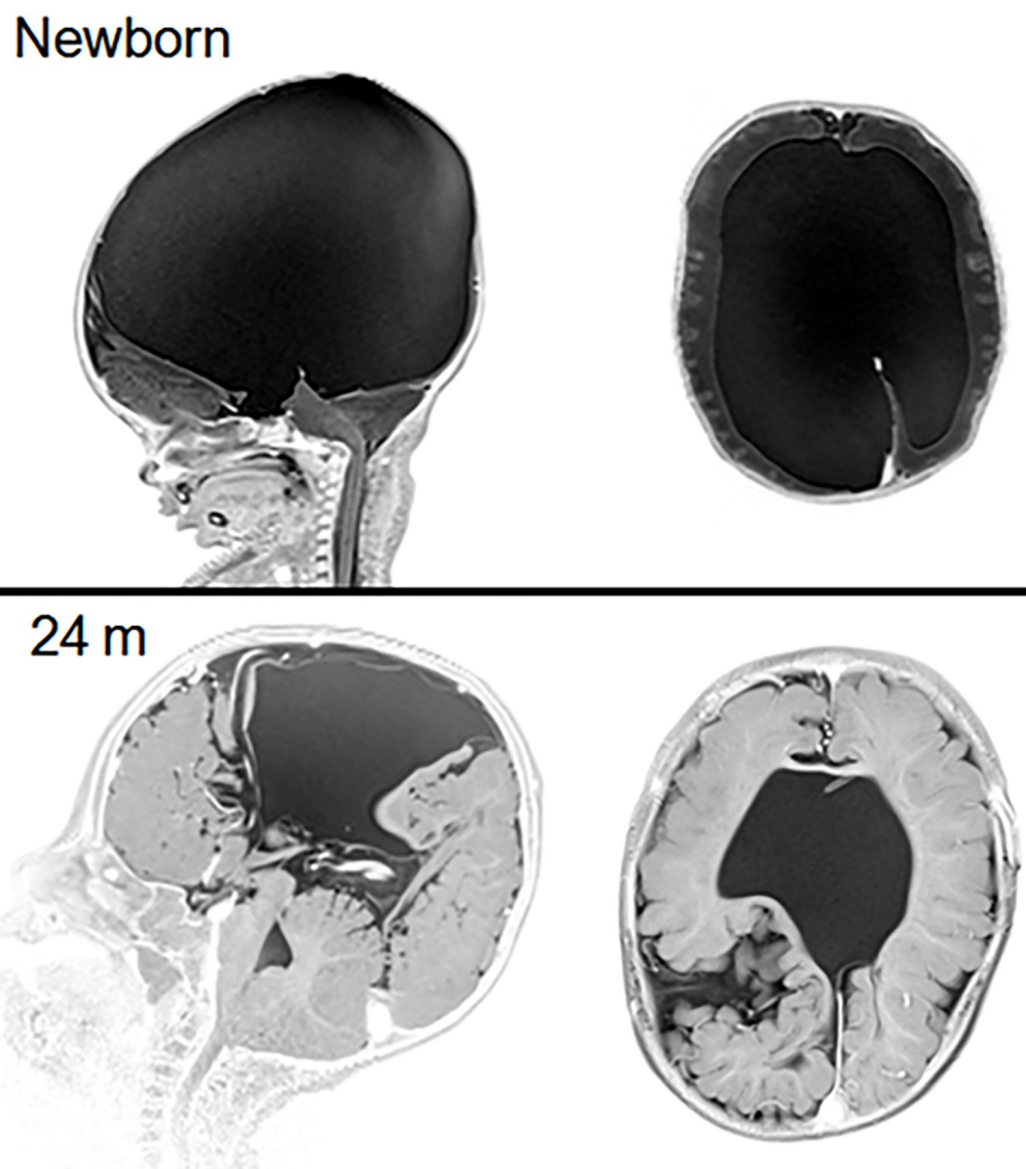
Representative example of boy with hydrocephalus due to aquaeductal stenosis. On the top, T2 sequence magnetic resonance imaging at birth showing the aquaeductal stenosis and the consequent hydrocephalus. On the bottom, T2 sequence magnetic resonance imaging control at 2 years old showing excellent cortical growth and normalization of the HC after VPS implantation. The pressure valve needed to be modified 8 times and the final pressure valve value amounted to 3 cm H_2_O (VPS: ventriculoperitoneal shunt; HC: head circumference).
